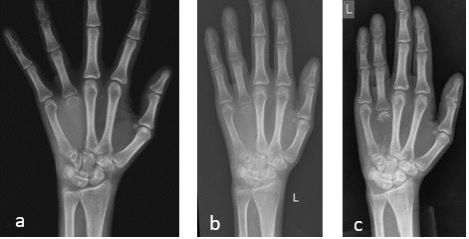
Left hand radiographs showing (a) lytic lesion with near complete destruction of the fourth metacarpal with progressive bone formation in (b) after 1 cycle of chemotherapy and (c) after 2 cycles of chemotherapy.
